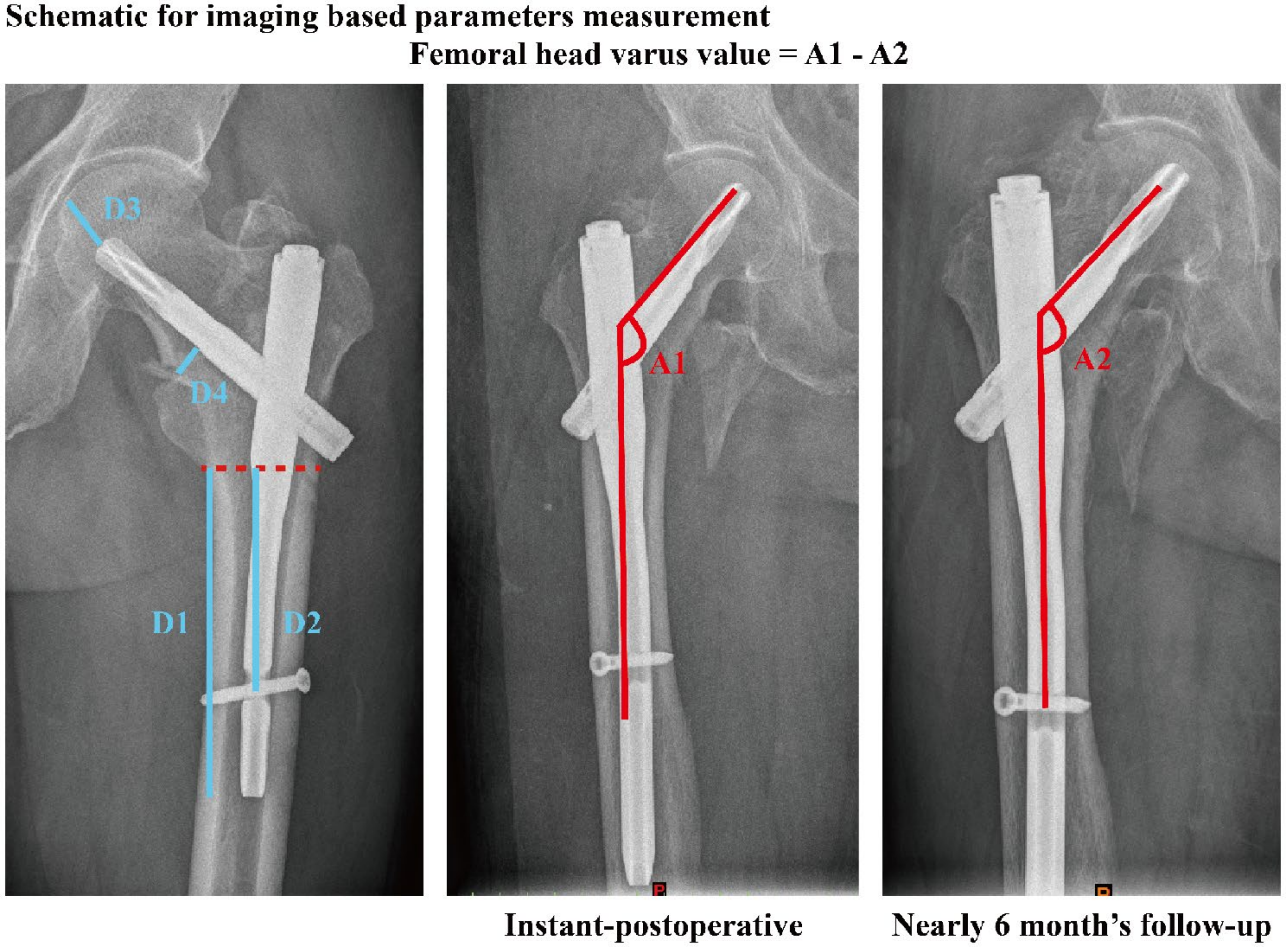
The measurement of imaging parameters: The length of the intramedullary nail in the femoral trunk (D1), the distance between the lesser trochanter and the distal locking screw (D2), and the femoral head varus values, TAD in the anterior-posterior radiography (D3), and the distance between femoral calcar and the anti-rotation blade (D4)
